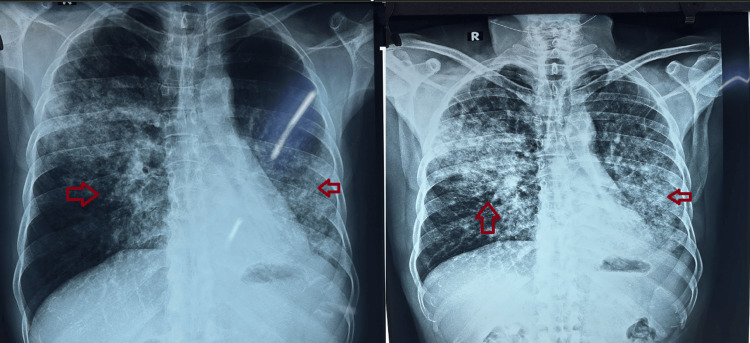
Chest radiograph (posteroanterior view) demonstrating bilateral heterogeneous opacities with predominant involvement of the left lower zone. Follow-up imaging shows persistent bilateral infiltrates with no significant resolution after anti-tubercular therapy.
